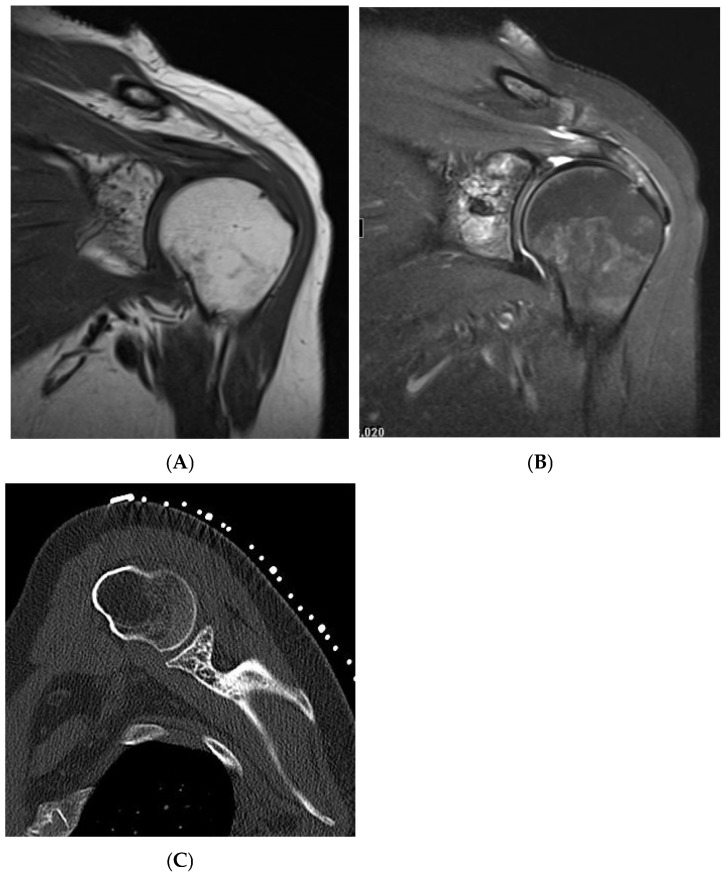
Haemangioma, left proximal humerus. (A) Coronal T1W MRI demonstrating a left glenoid predominantly hyperintense lesion with speckled low-intensity areas representing mineralization. (B) Coronal STIR MRI. (C) Axial CT of the left glenoid demonstrating “polka-dot” sign—low-density fat lesion with interspersed foci of mineralization.
